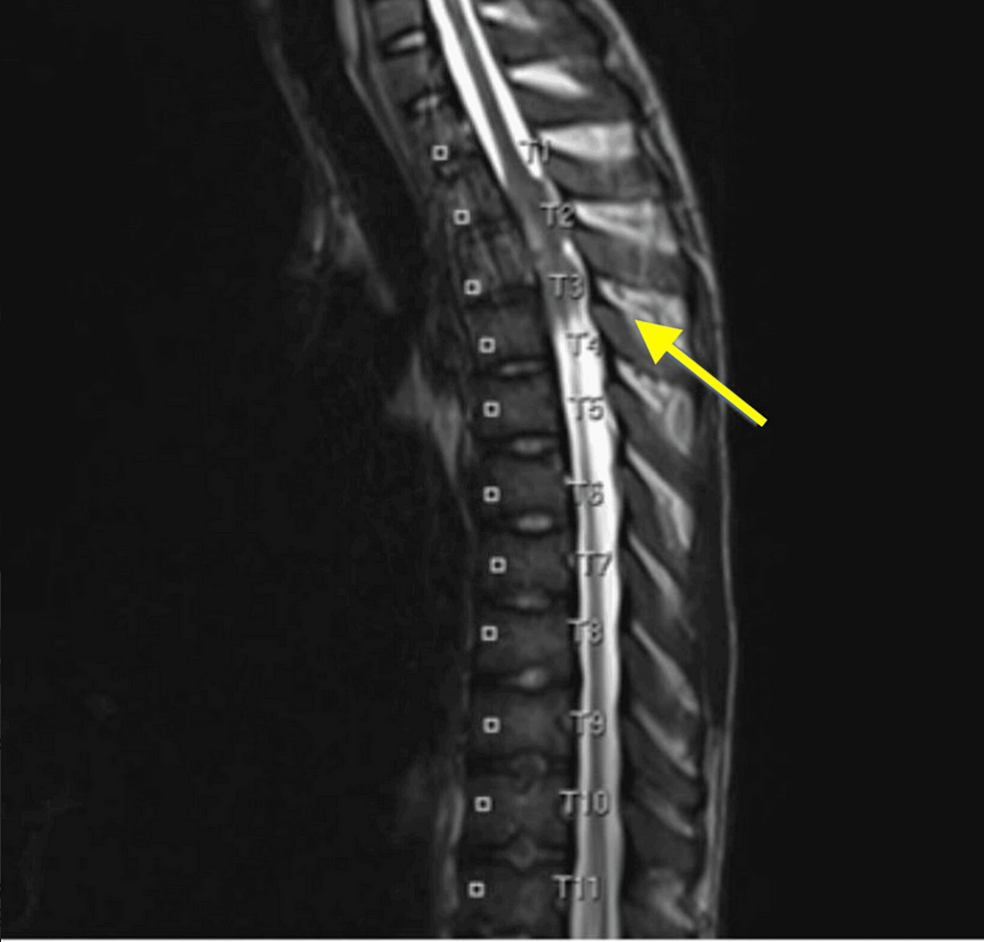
Magnetic resonance imaging (MRI) scan showing metastatic spinal cord compression.
